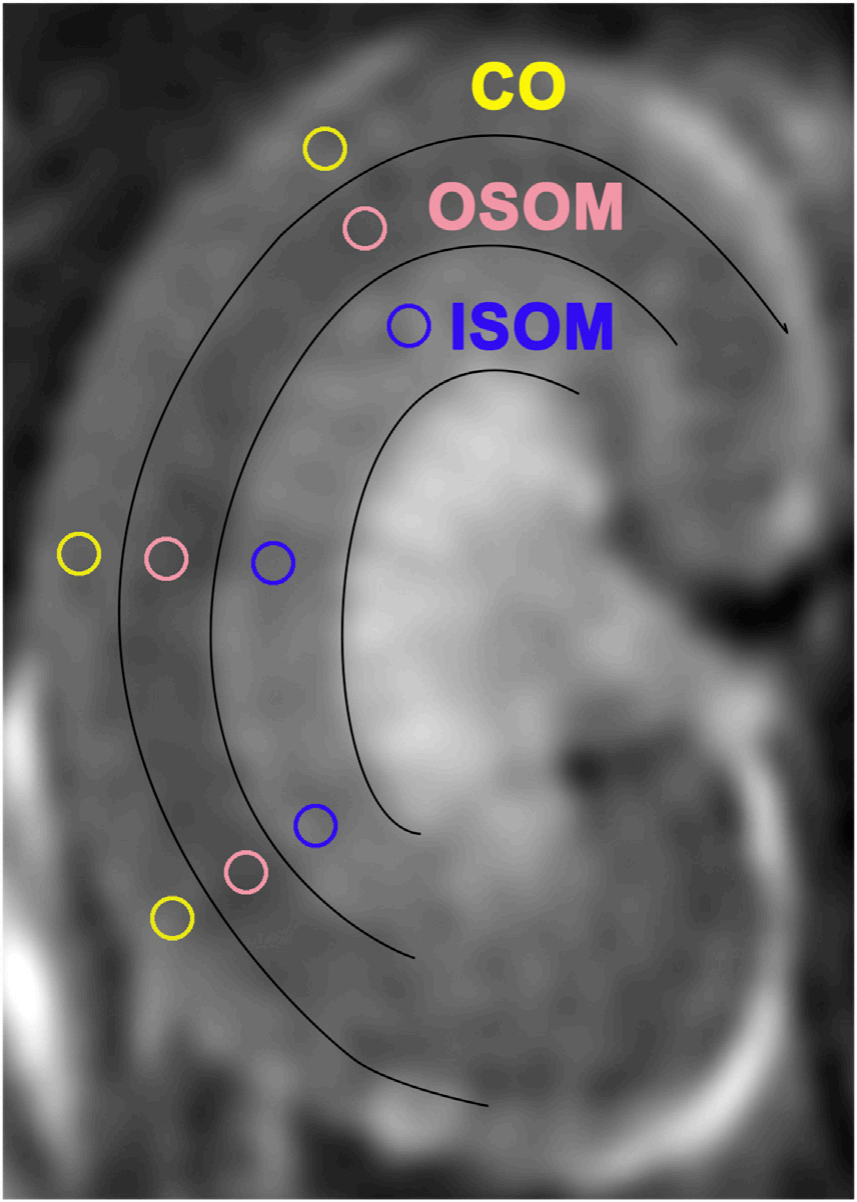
Regions of interest (ROIs) in three sectors of T2-weighted images. ISOM: inner stripe of the outer medulla; CO: cortex; OSOM: outer stripe of the outer medulla.
